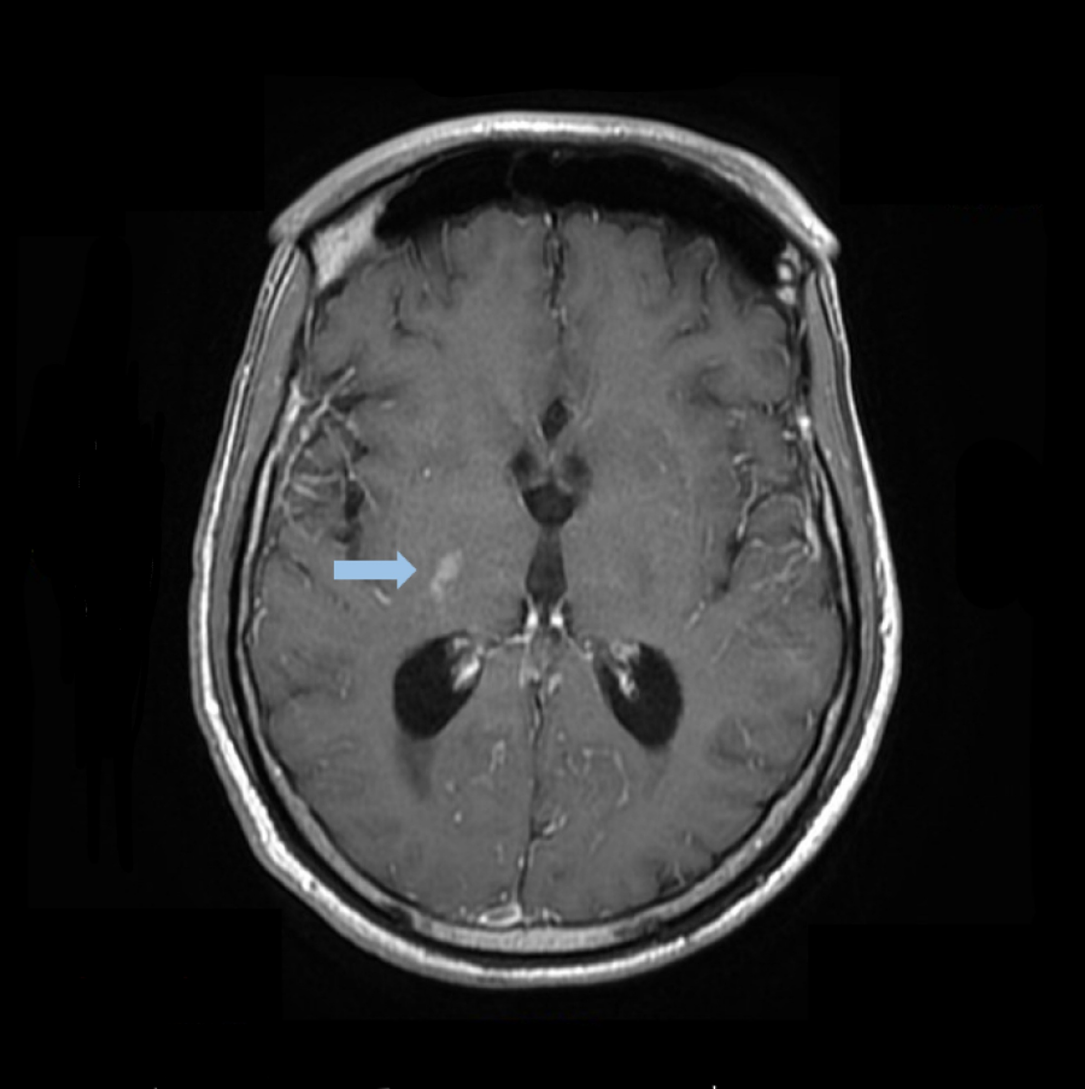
Magnetic resonance images of the head with contrast showing right basal ganglia lesion Blue arrow indicates cryptococcomas lesion.
